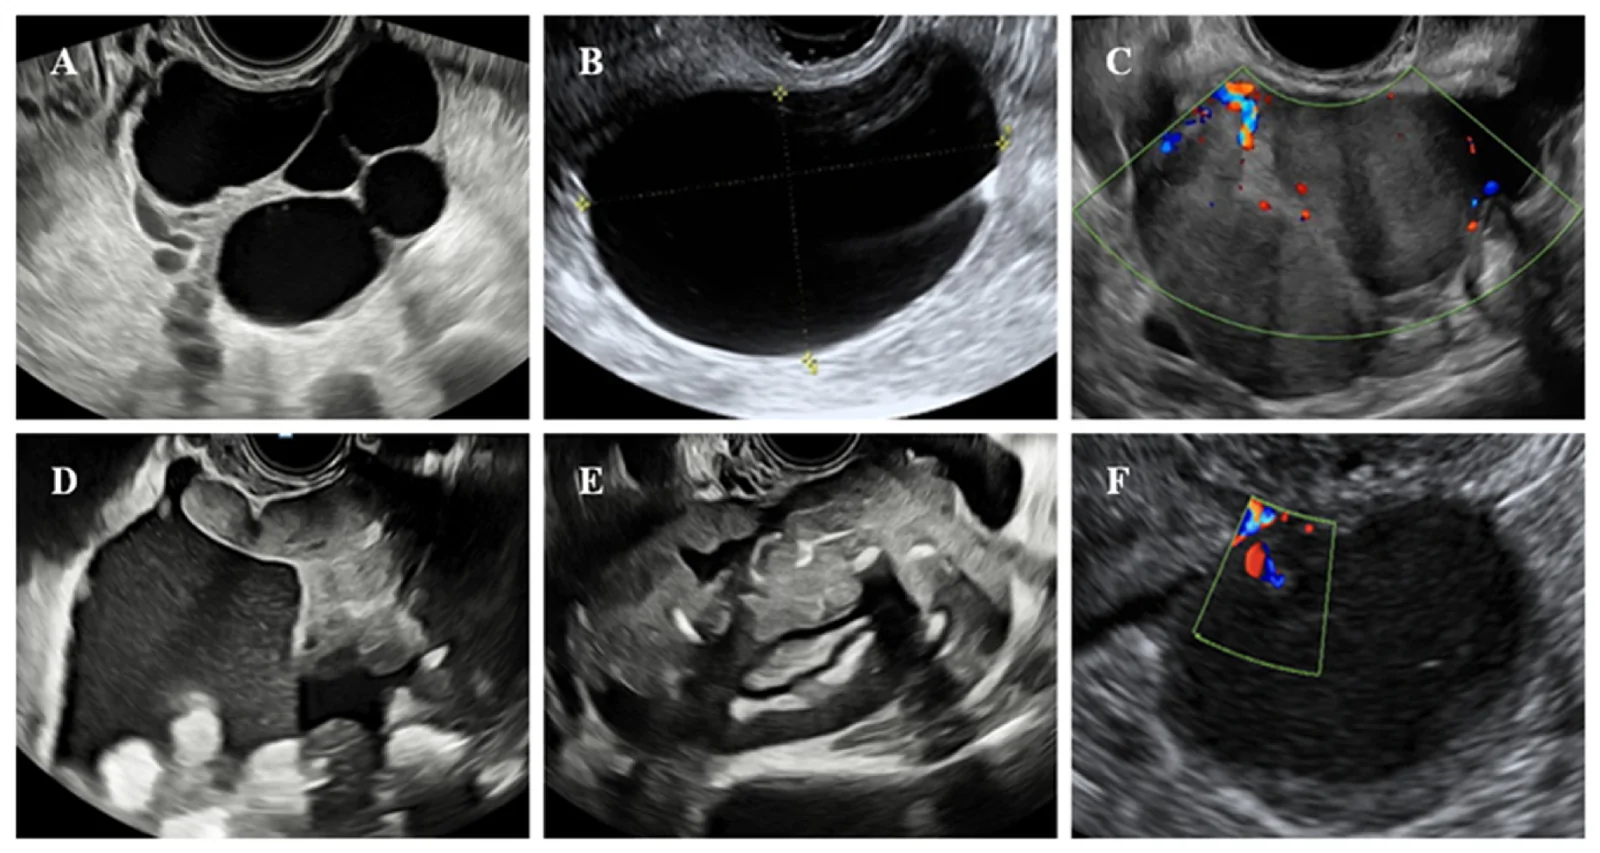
Predefined and additional ultrasound parameters selected to describe the adnexal masses, according to IOTA terminology. (A–C) show examples of predefined parameters, respectively morphology ((A), e.g., multilocular cyst), size (B), and vascularization of the lesion (C), used in all of the ChatGPT configurations proposed. (D) shows the presence of ascites, an additional clinical and ultrasound parameter considered in ChatGPT C configuration. (E) represents an example of acoustic shadows, an additional ultrasound parameter considered in ChatGPT D configuration. (F) represents a vascularized papilla, an additional ultrasound parameter considered in ChatGPT configuration E.
